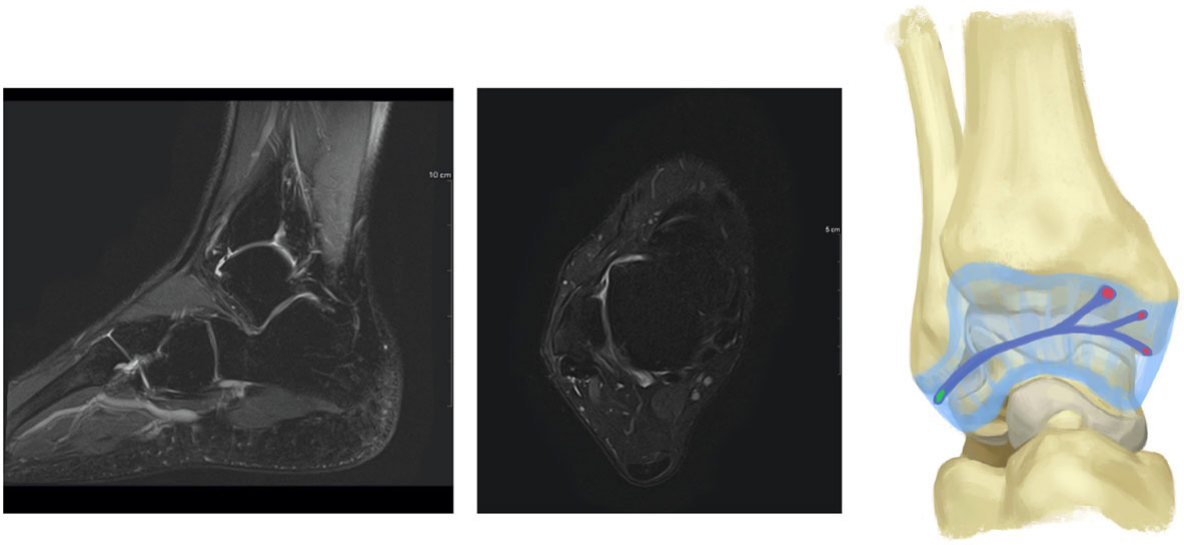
Sagittal and axial MRI views, and the shape of the fibrous band in patient 4
